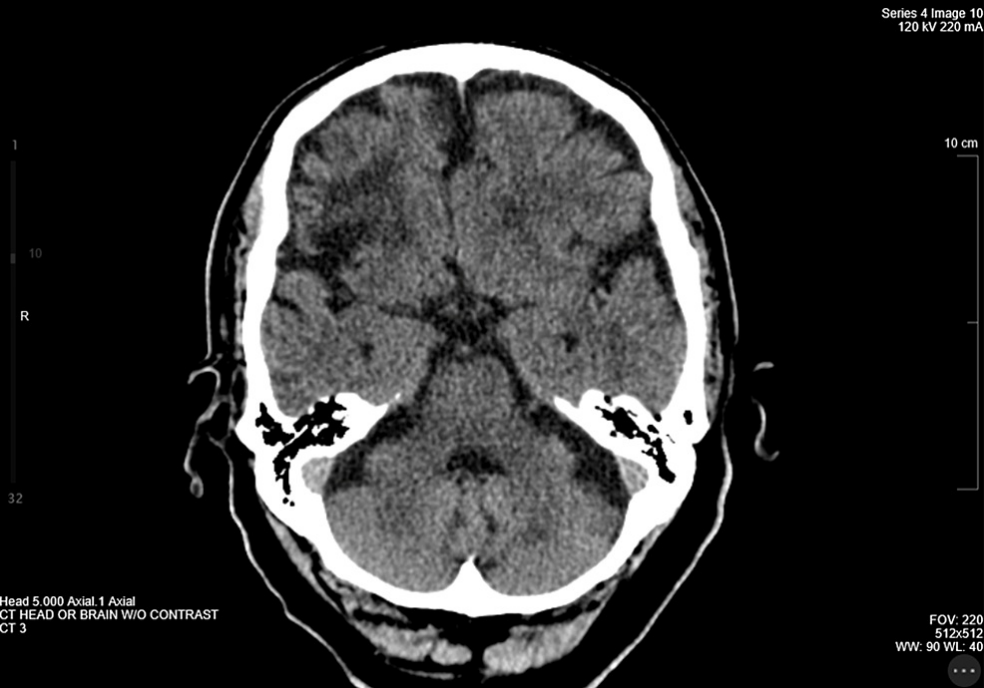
Posterior Fossa CT Scan Findings
